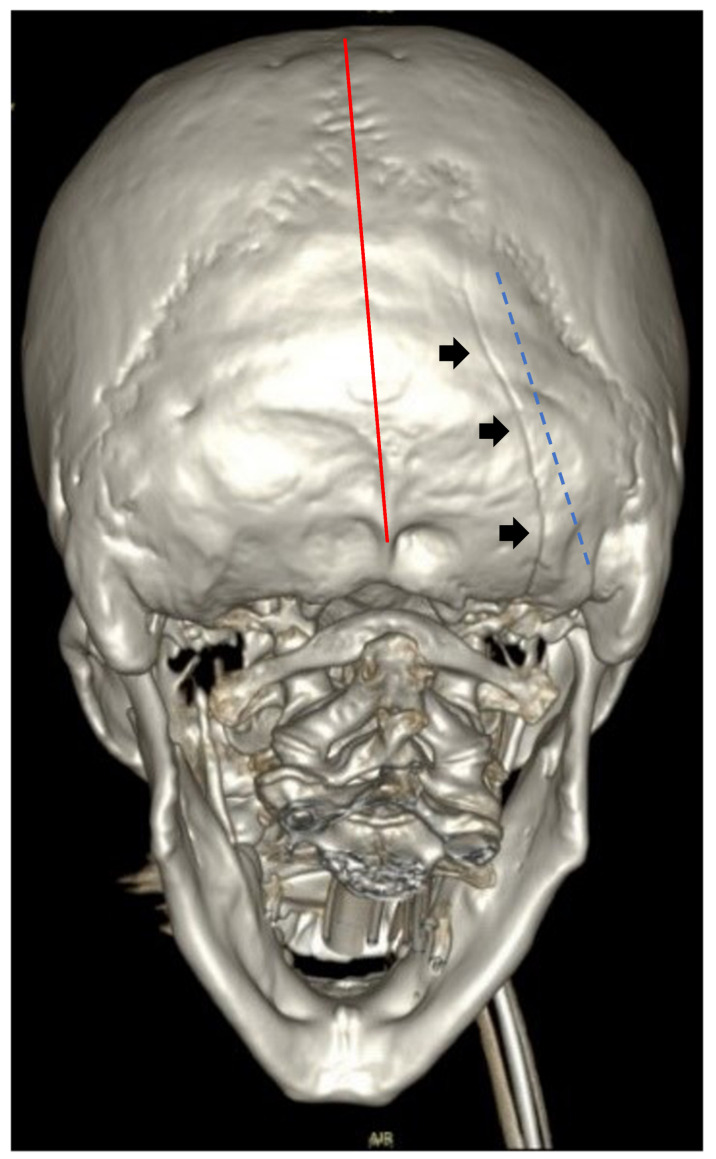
Three-dimensional reconstruction of the patient’s fracture line (dark arrows) based on CT images. Red line corresponds to the sagittal plane; blue dotted line highlights the oblique orientation of the fracture.
